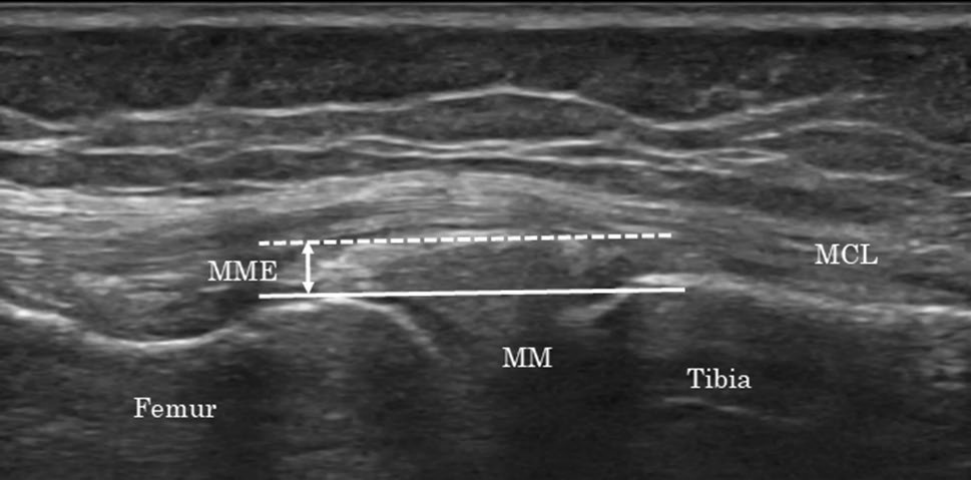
Methods for evaluating MME via ultrasonography using longitudinal sections parallel to the MCL. *MCL* medial collateral ligament, *MME* medial meniscal extrusion, *MM* medial meniscus.
